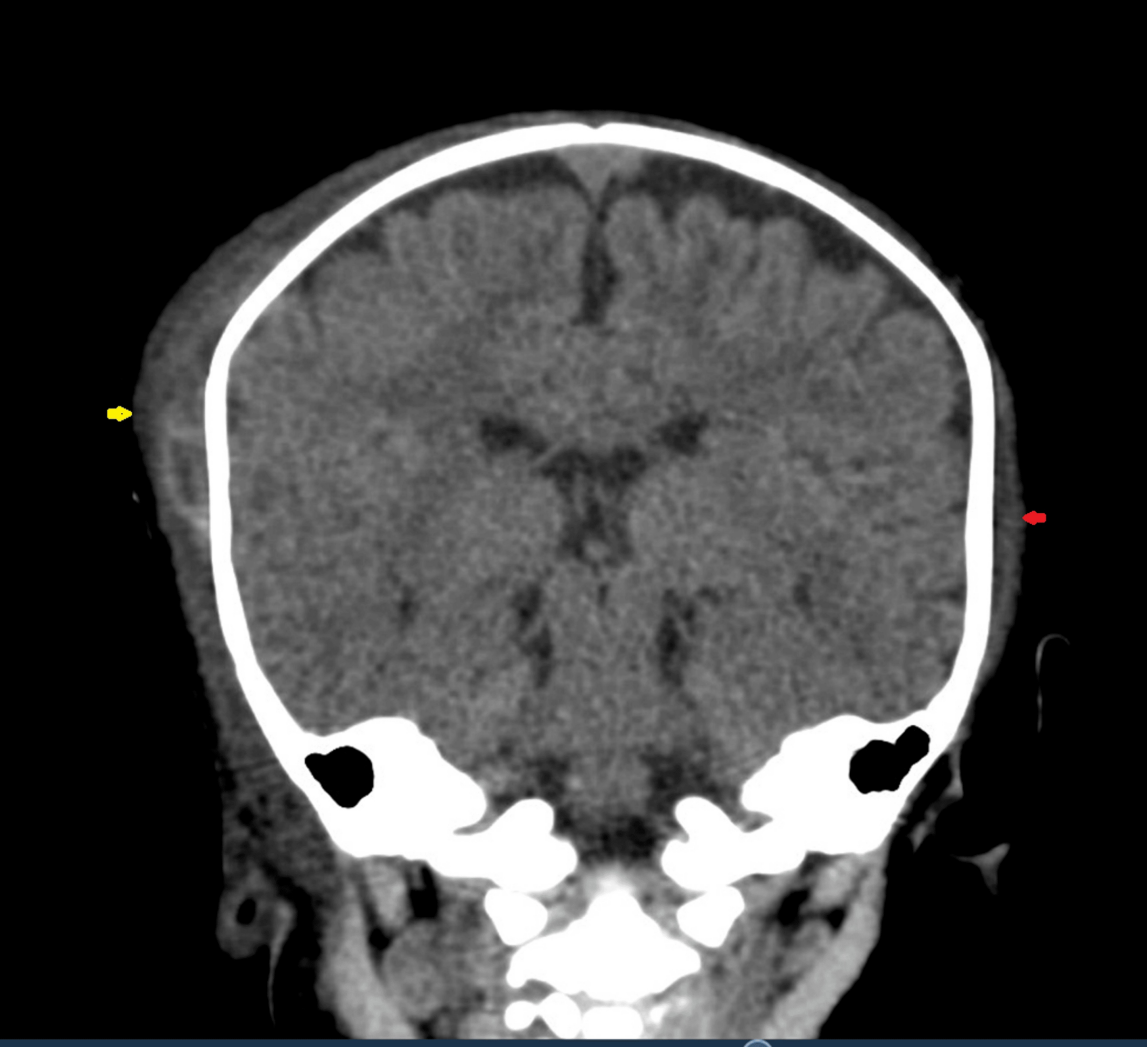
CT of the brain (coronal plane) showing right-sided subgaleal hematoma (yellow arrow) with involvement of the opposite side (red arrow).
